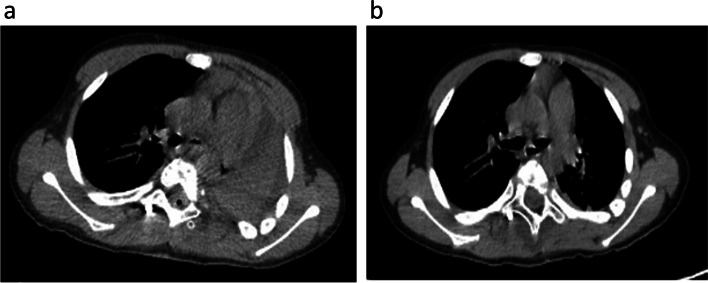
A 9-year-old male boy developed complete left atelasis and bronchoscopy was needed for lung expansion. He had C7 and T2/3 hemivertebra resection and posterior fusion. He developed tachypena and tachycardia on postoperative day 1. Physical examination showed asymmetric thoraci movement, absent left breath sound. And chest CT showed complete left pulmonary atelasis and pleura effusion (See Figure a). He had brochoscopy under general anesthesia and bronchoscopy revealed sputum completely occluded left main bronchus. Figure b showd left lung expanded after the sputum plug was removed
